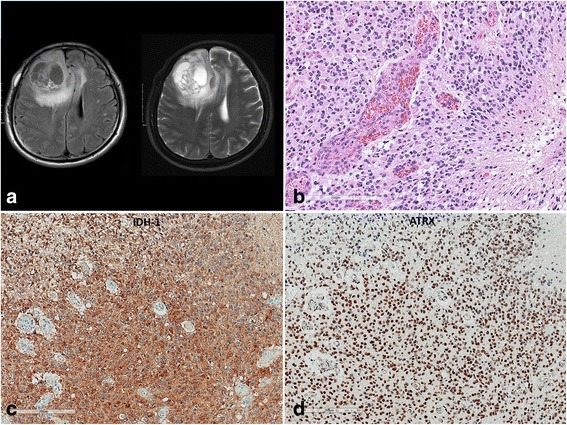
a) MRI showing a solid and cystic mass involving the right frontal lobe with diffuse increased T2 signal intensity in the right frontal lobe, insula, and corpus callosum genu with subfalcine herniation on the left side. b) The tumor is indicative of glioblastoma by the presence of necrosis and microvascular proliferation. c, d) Tumor cells are positive for IDH-1 (H09), R132H-mutant, and retained expression of ATRX
